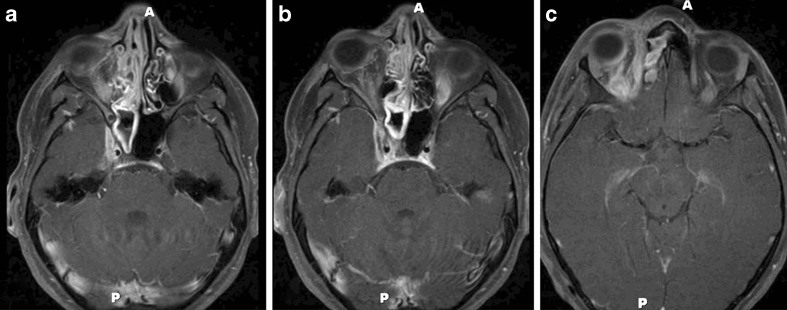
MRI of head and orbits performed with and without contrast. Serial non-contrast axial images showing worsening right orbital cellulitis and enlargement of the right cavernous sinus (a–c)
